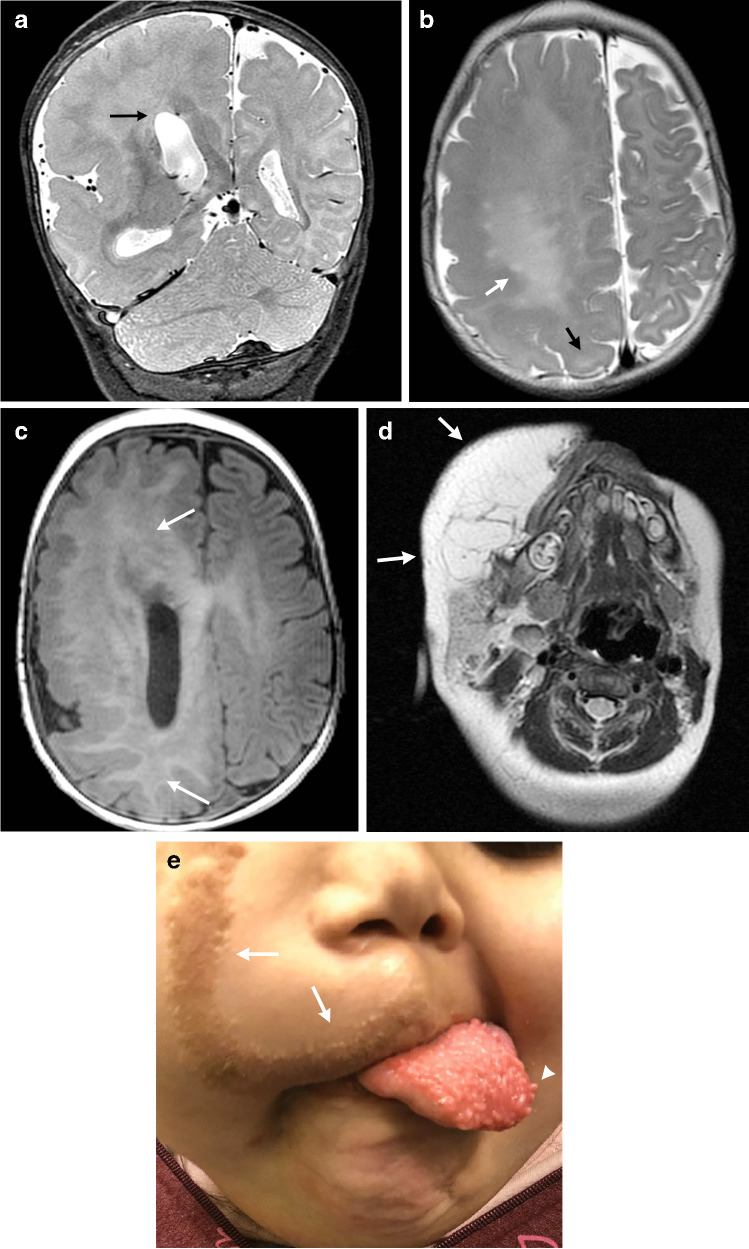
CLOVES (*c*ongenital *l*ipomatous *o*vergrowth, *v*ascular anomalies, *e*pidermal nevi, and *s*coliosis/spinal deformities) syndrome in a 4-month-old girl with a genetically confirmed *PIK3CA* mutation with hemimegalencephaly and facial overgrowth. a A coronal T2-weighted image shows right hemimegalencephaly in the cerebrum and cerebellum. The right lateral ventricular body is large and superiorly directed (*arrow*). b An axial T2-weighted image shows increased signal within the white matter (*white arrow*). Pachygyria is noted (*black arrow*). c An axial spoiled gradient recalled echo image shows increased signal throughout the white matter (*arrows*). d An axial T2-weighted image shows substantial lipomatous overgrowth in the right face (*arrows*). e A clinical photograph at 5 years of age shows the corresponding right facial overgrowth. Linear epidermal nevi are seen (*arrows*). The tongue is also enlarged with verrucous papules (*arrowhead*). Consent for the photograph was obtained from the patient’s legal guardian
